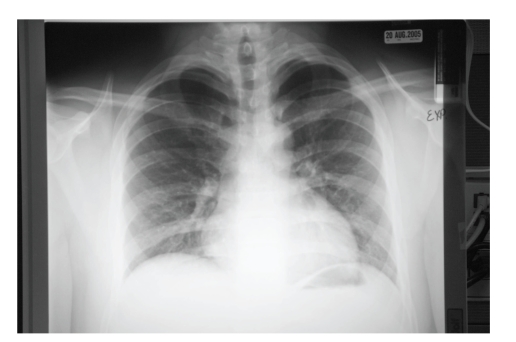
Follow-up chest X-ray, expiratory view, two weeks later, shows resolution of previous findings.
